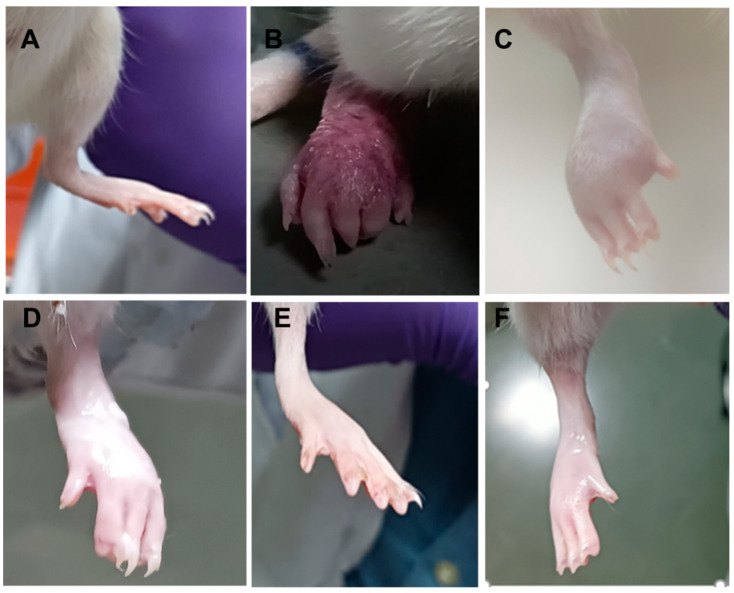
Representative images of FCA injected hind paws after 28 days of treatment with various formulations: (A) normal; (B) AIA; (C) CF018P emulgel; (D) FDG; (E) CF018 emulgel; (F) MFD.
